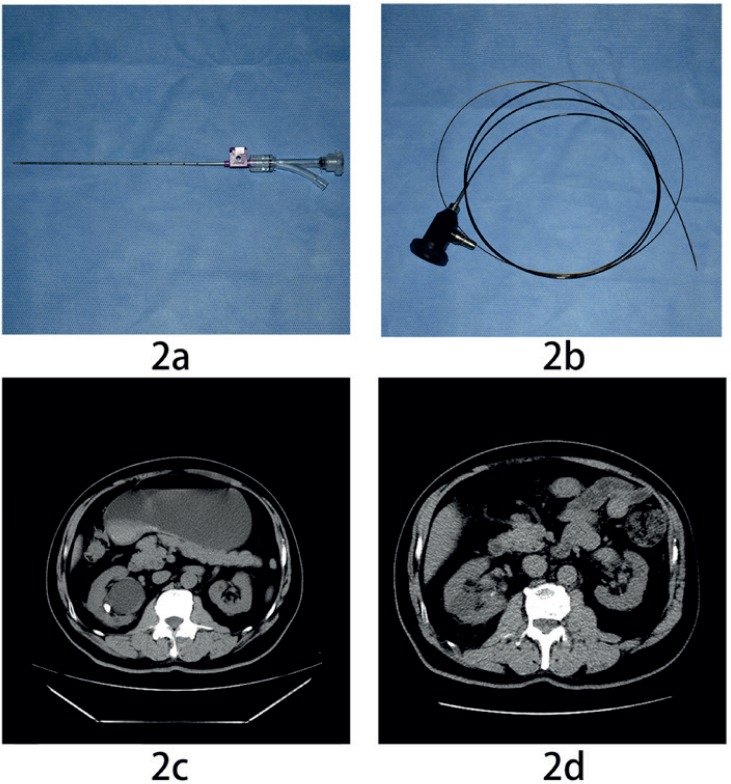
a) One adapter attached to the tip of the outer shaft; 2b) The 0.7-mm fiber-endoscope of the flexible ureterorenoscope; 2c) preoperative CT showing the right parapelvic cyst and renal stone; 2d) postoperative CT showing the absence of cysts and stones.
